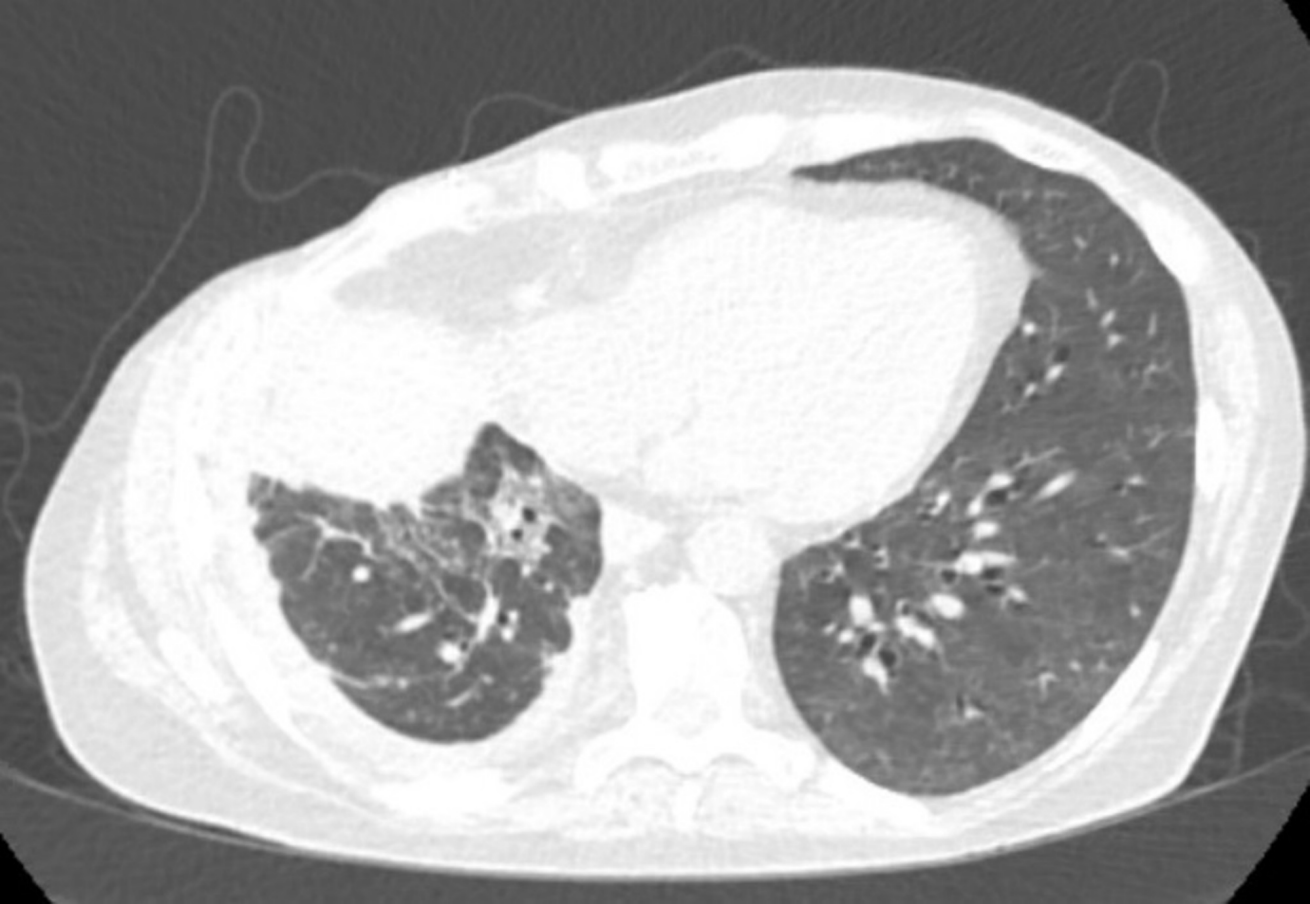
Subsequent computed tomography (CT) scan of the chest. Subsequent CT scan of the chest showing marked improvement in the ground glass opacities in the left lung, although the primary lung mass has increased in size.
